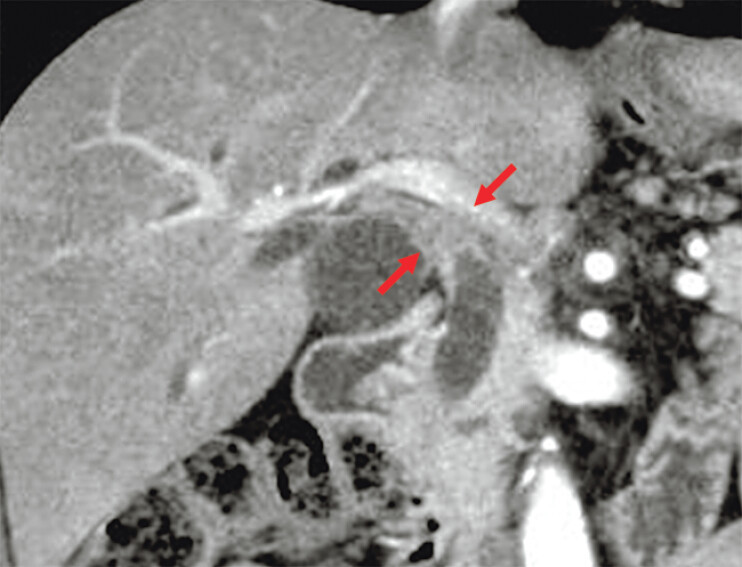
Computed tomogram showing the biliary stricture caused by bile duct cancer in the hilar bile duct of a 68-year-old man (arrows).
